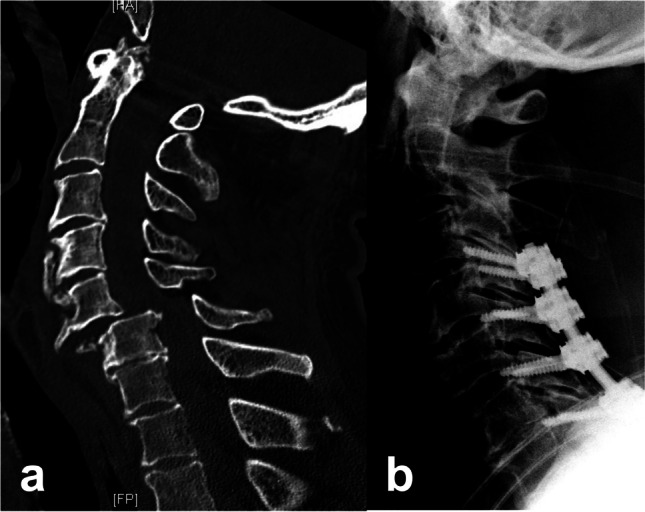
82-year male patient presenting with progressive motor weakness noted in the triceps, and poor hand response. His lower extremities were 1/5. a Emergency CT showing fracture dislocation at C5–C6 with anterior and posterior ligamentous disruption. b Patient underwent fracture reduction and posterior instrumentation from C4 to C7
